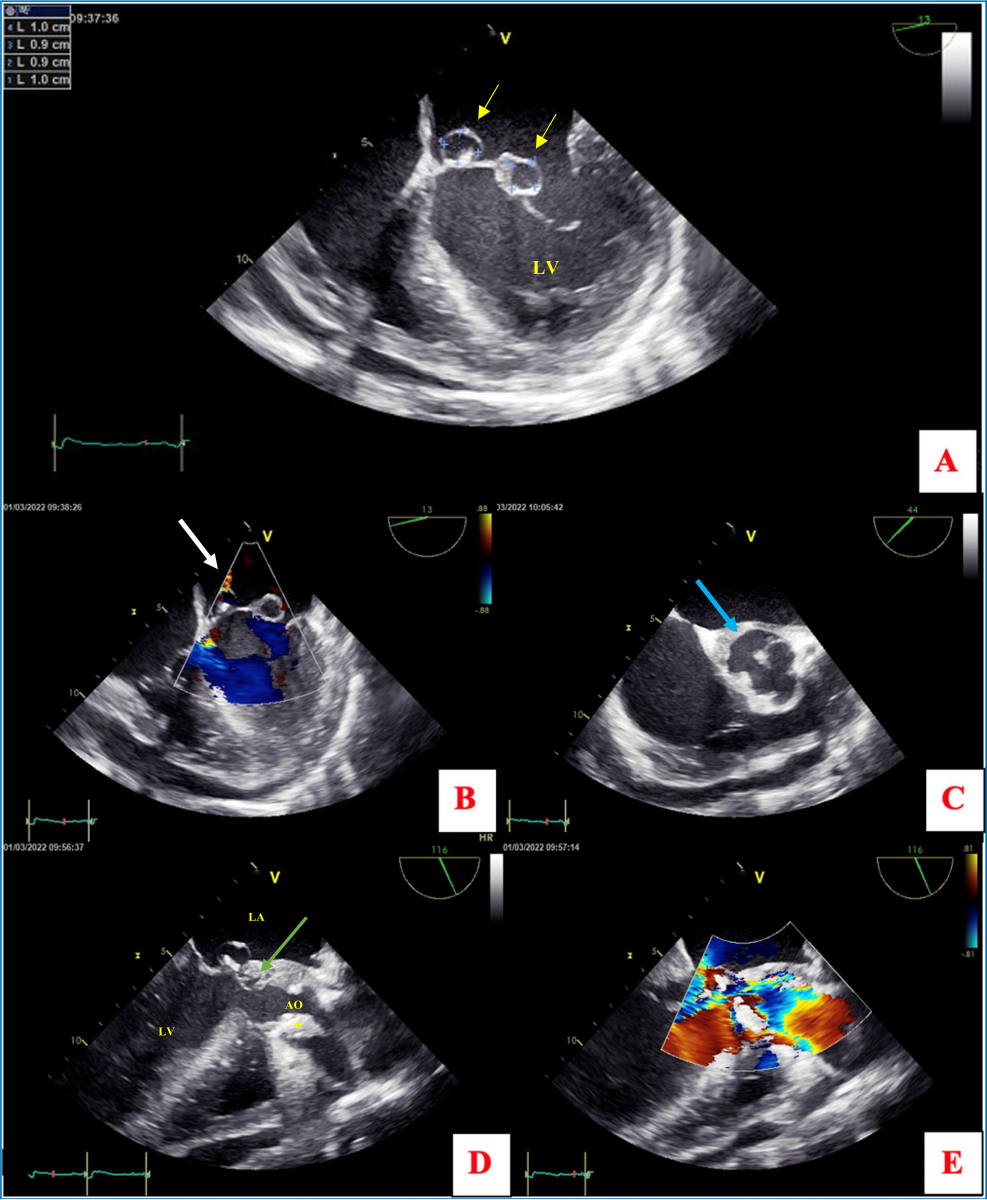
Transesophageal echocardiography (TEE) A Four-chamber mid-esophageal TEE showing two abnormal ring-like structures on the mitral valve compatible with aneurysms (yellow arrows). B Color Doppler analysis showing a turbulent flow through a small orifice within the medial anterior mitral valve leaflet aneurysm related to a mitral regurgitation (white arrow) C Mid-esophageal TEE short axis view showing a bicuspid aortic valve with thickening of the aortic-mitral fibrous trigone indicating an aortic root abscess (blue arrow) D three-chamber mid-esophageal TEE demonstrates an anterior mitral leaflet aneurysms juxtaposed to a prominent aortic root abscess (green arrow) and vegetation of the right coronary valve (*) E Color Doppler study showed a significant aortic regurgitation with an eccentric jet directed toward the anterior mitral leaflet aneurysm. LA = left atrium, Ao = ascending aorta, LV = left ventricle
